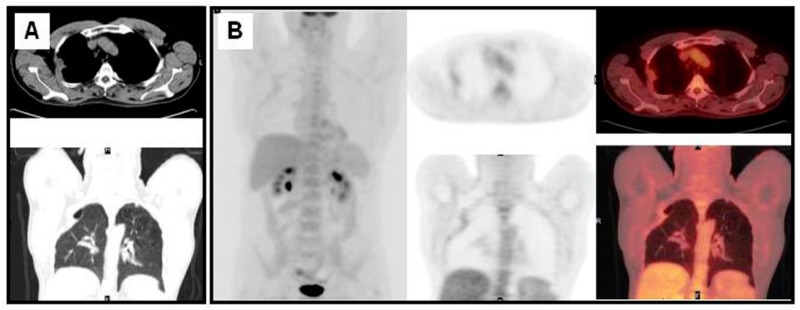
Patient with undifferentiated carcinoma of the right lung surgically treated seven years before followed by radiotherapy. In a context of pain and functional impotence of the right shoulder, a CT showed a suspicious image in the medial aspect of the lung near the right axilla and structural changes due to the previous surgery (A); ^18^F-FDG PET/CT ruled out recurrence (B).
